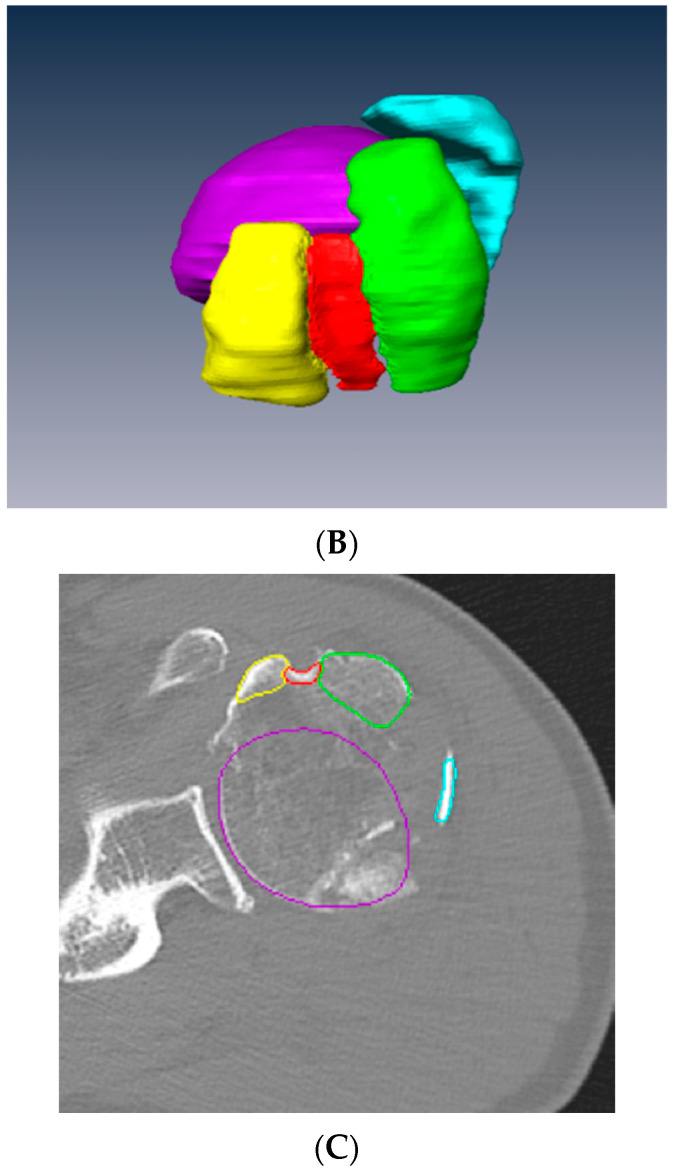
Segmentation of fractured fragments using AMIRA^®^ software. (A) 3D mesh posterior view; (B) 3D mesh anterior view; (C) Fragment boundaries were contoured by hand on every other axial CT section. Here, the humeral head is in purple, the greater tuberosity in green (unfractured) and blue (posterior fractured portion), and the lesser tuberosity in yellow.
